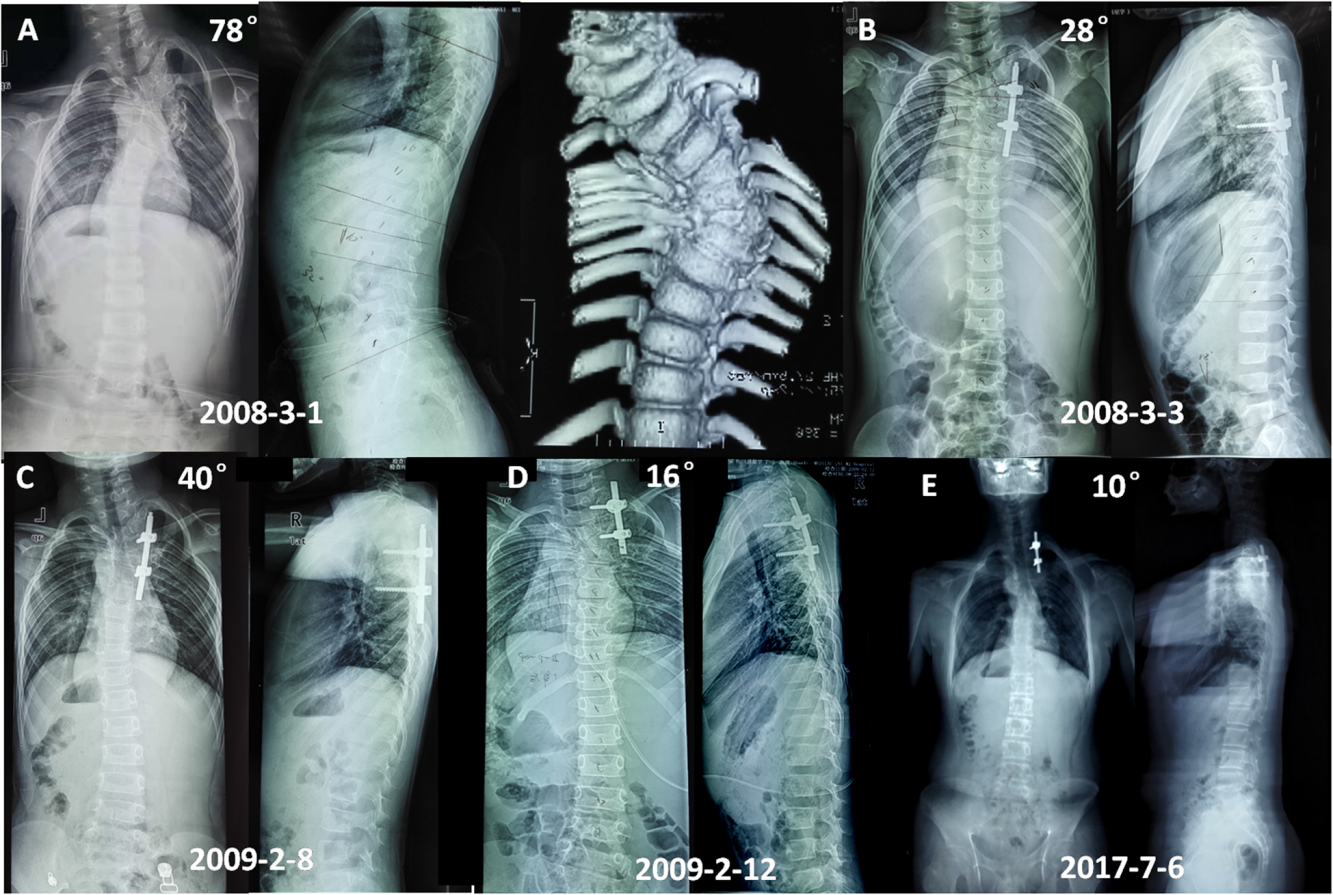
An 8 years old boy with complex congenital scoliosis. Radiographs and 3D CT showed T5 semi-segmented hemivertebra, T2-4 bloc vertebra and concave fused ribs (A). He underwent T5 HVR with unilateral short fusion at initial surgery (B). Second surgery including T2 Y-shaped osteotomy was performed after 11 months because proximal curve progression. Radiographs images obtained preoperatively, postoperatively and at the latest follow-up 101 months later (C,D,E)
